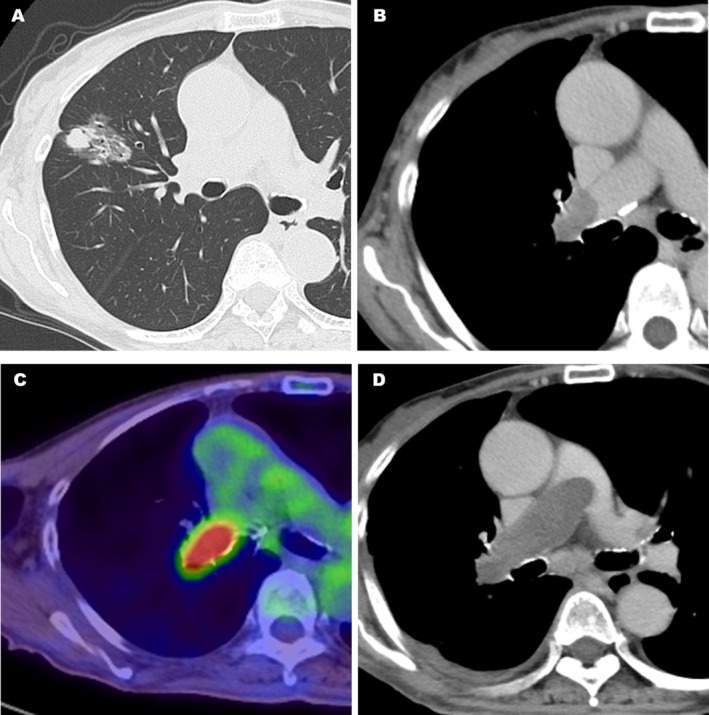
(A) Chest CT at initial presentation showing a 20‐mm nodule in the right upper lobe, consistent with primary lung adenocarcinoma. (B) Follow‐up chest CT 2 years after surgical resection demonstrating a newly appeared mediastinal mass adjacent to the right main pulmonary artery, initially interpreted as lymph node metastasis. (C) PET‐CT showing intense FDG uptake in the mediastinal mass. (D) CT obtained at disease progression confirming intraluminal extension of the tumour from the right main pulmonary artery toward the bifurcation, establishing the intravascular localization of the tumour.
